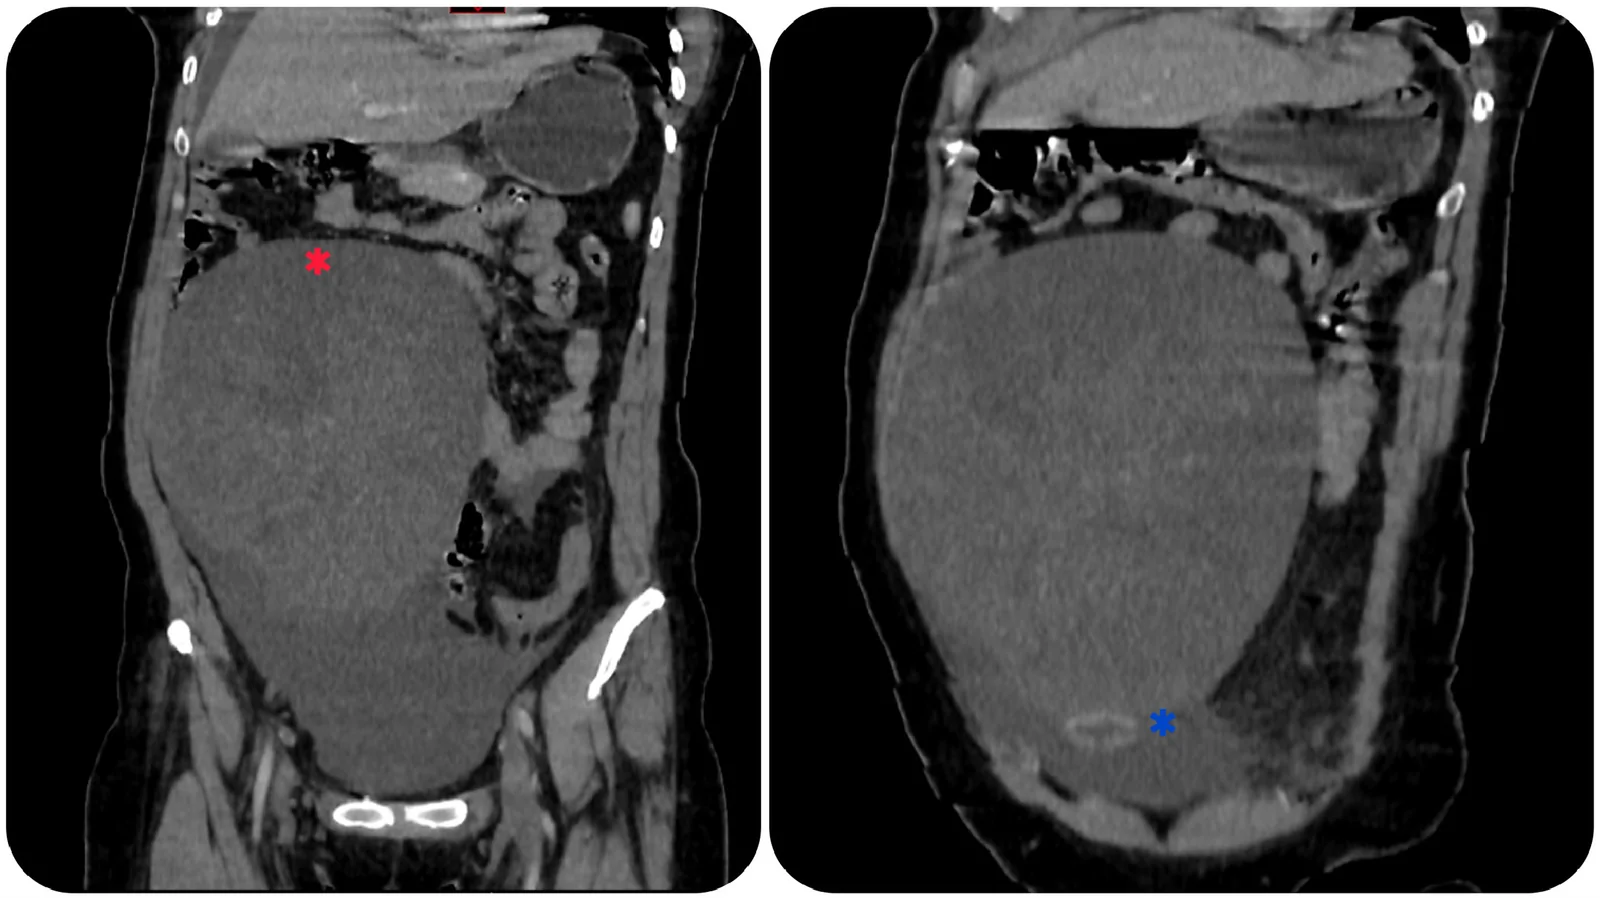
Coronal contrast-enhanced CT reconstructions showing the cranial (red asterisk) and caudal (bleu asterisk) extent of the ovarian mass and its relationship with adjacent abdominal structures.
